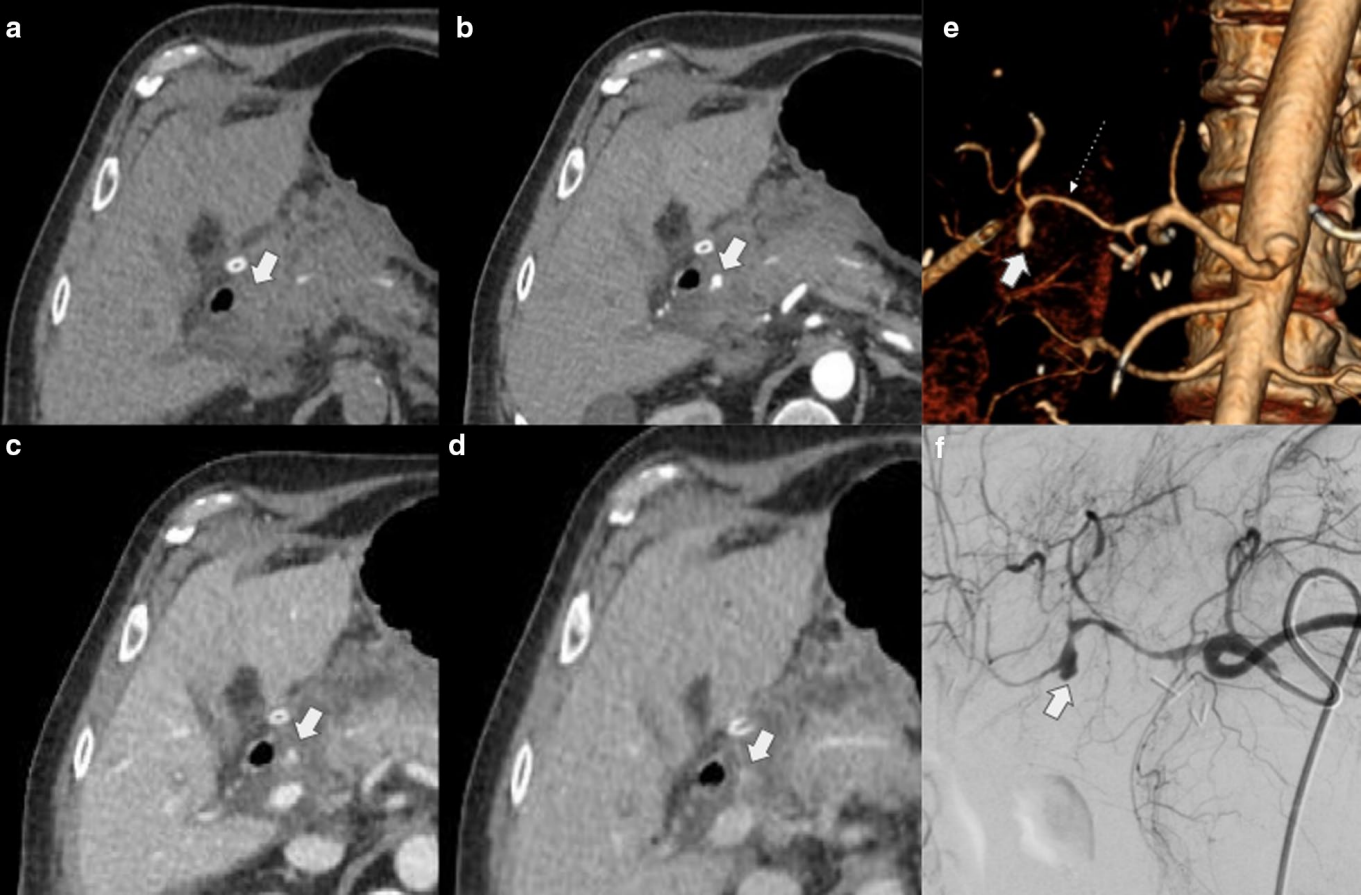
Right hepatic pseudoaneurysm. 78-year-old male patient with a history of pancreaticoduodenectomy with right hepatic artery reconstruction for pancreatic adenocarcinoma under study due to hematocrit decrease. (a, b, c, d) Multiphase CT shows a focus of focal enhancement in arterial phase in the surgical bed (arrow in b), which persists in post-arterial phases (big arrows in c and d). (e) 3D reconstruction shows saccular dilatation (arrow) dependence of the right branch of the hepatic artery (dotted arrow). (f) Digital angiography confirms the diagnosis of pseudoaneurysm
